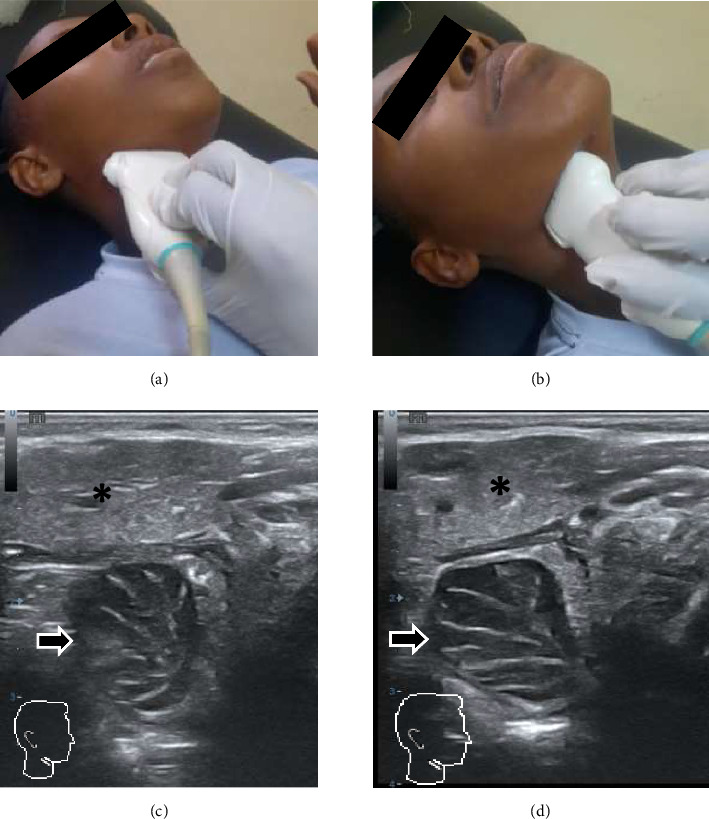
Measurement technique and transcutaneous submandibular sonographic views. Tonsil US performed via submandibular approach on a 10-year-old study participant. The correct placement of the linear transducer in the transverse plane with the probe marker facing the patient's right ear (a) and in the longitudinal plane underneath the mandible (b) are shown. Gray-scale image in transverse (c) and longitudinal planes (d) show the right palatine tonsil (arrows in (c) and (d)) as an ovoid hypoechoic structure located deep to submandibular gland (asterisk in (c) and (d)).
